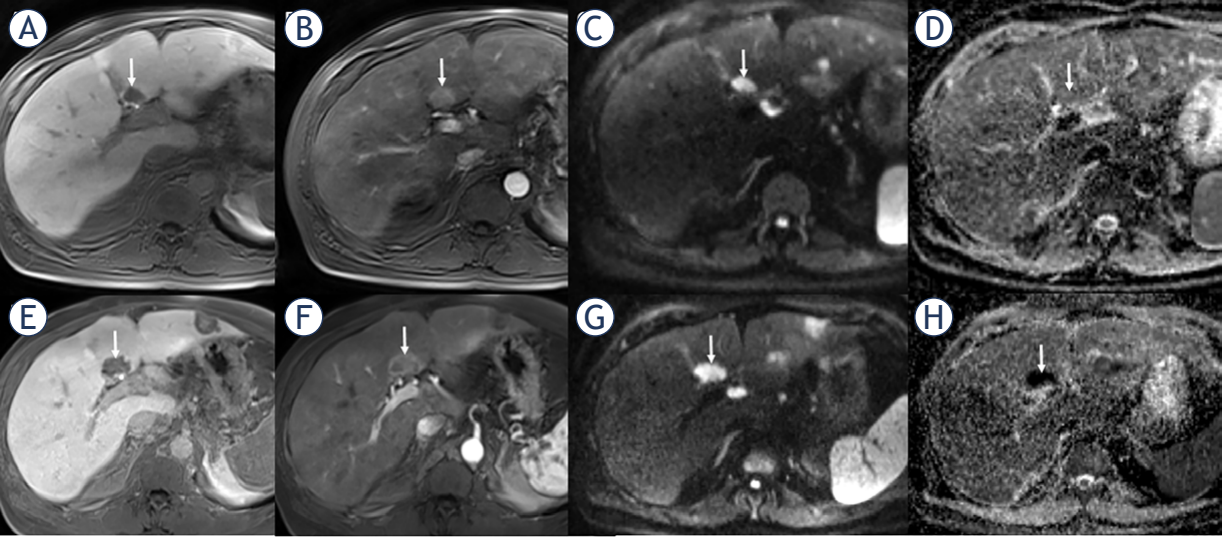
A 56-year-old man with liver metastasis of pancreatic NET classified as nonresponder with a PFS of 3 months. The baseline axial contrast-enhanced T1- weighted image (hepatobiliary phase) (A) shows a hypointense lesion (arrow) in segment 4A. The metastasis shows a strong artrerial enhancement (B) and restricted diffusion (arrow) with high signal on axial DW-MR image b = 800 s/mm^2^
